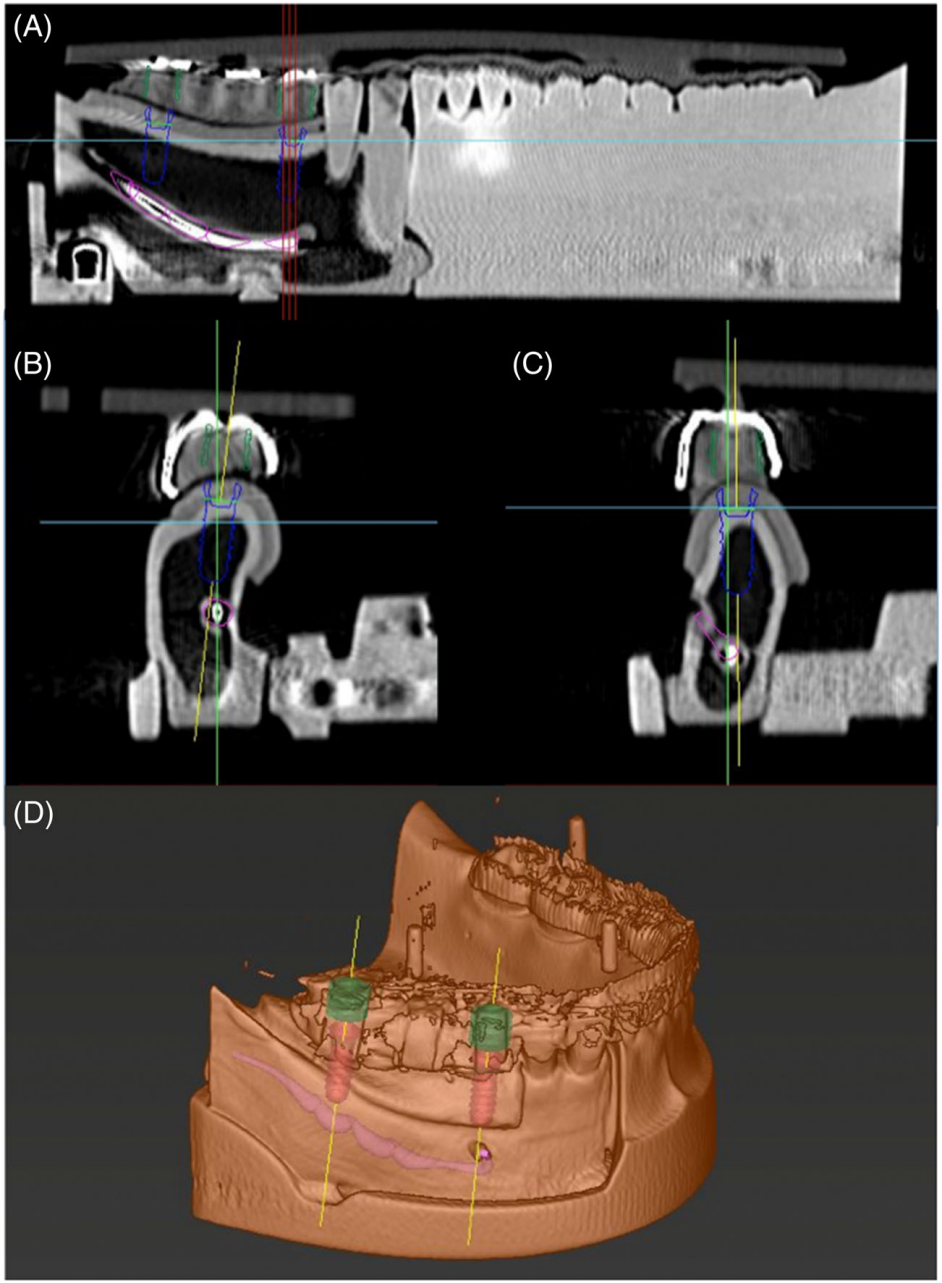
Virtual planning of implant position on the 45 and 47 regions from preoperative CT. (A) Panoramic image on the 45 and 47 regions. (B) Cross‐sectional image on the 47 region. (C) Cross sectional image on the 45 region. (D) 3D image of virtual planning.
